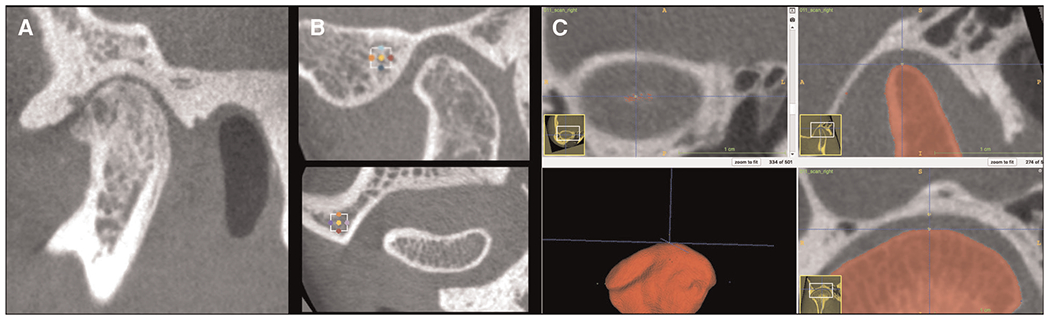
TMJ imaging protocol. (A) Small FOV hr-CBCT scans for TMJ imaging features analysis. Note that marked bone destruction is seen in the condyle and the articular fossa also shows erosion. (B) Volumes of interest (VOIs) in the lateral portion of the articular fossa and in the articular eminence regions. (C) Superior condylar-to-fossa distance as indicated by the blue axis line seen in the coronal and sagittal views.
